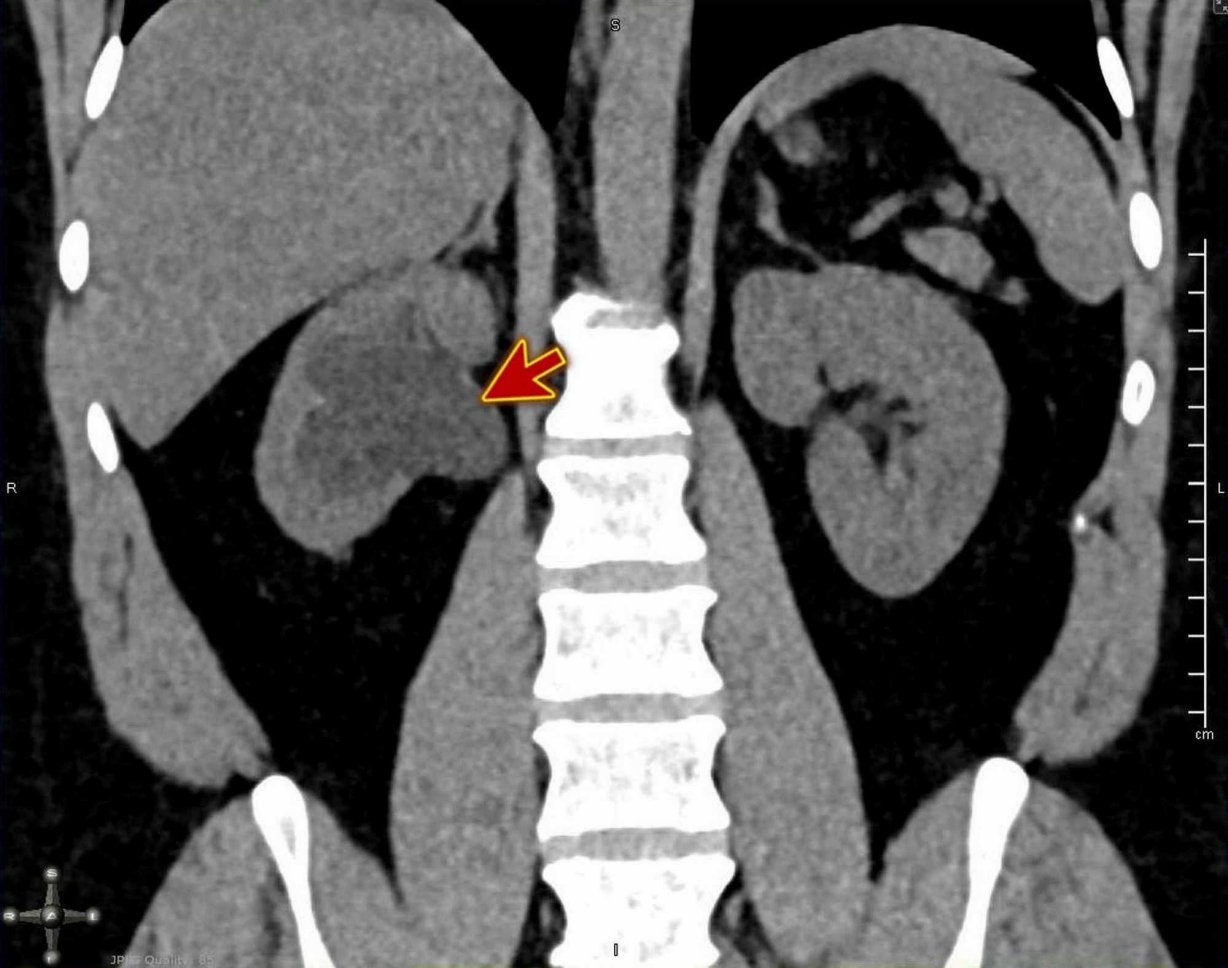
CT KUB showing right moderate to severe hydronephrosis (red arrow). CT KUB, computed tomography of the kidney and the urinary bladder
